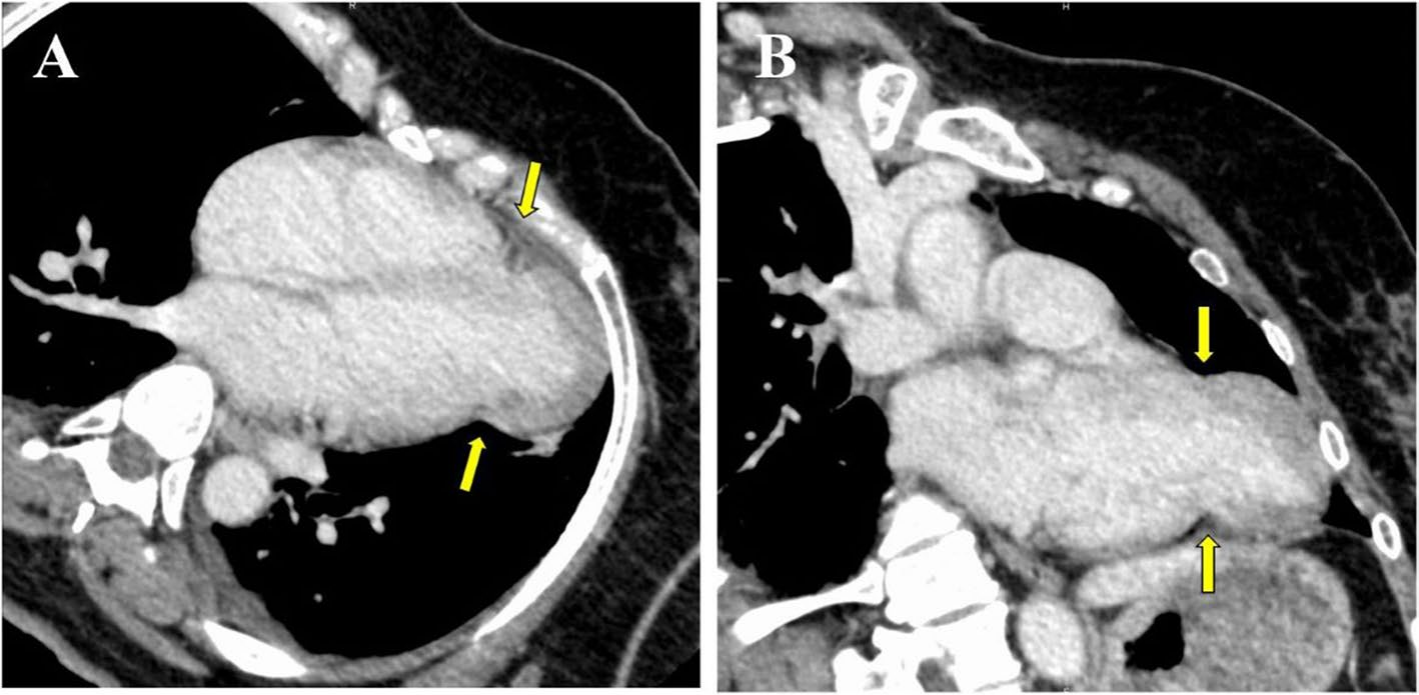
Contrast-enhanced computed tomography of the chest. Axial (A) and sagittal (B) views showing cardiac herniation into the left thoracic cavity from the mid-left ventricle to the apex (yellow arrow)
